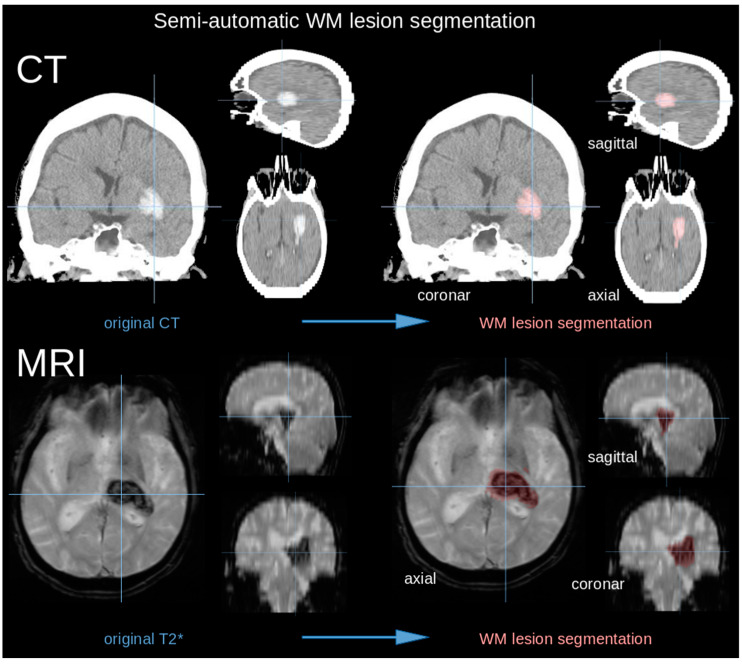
(Upper panel) CT scan in coronar, axial, and sagittal slice representation (see crosshairs) with colored infarct area (right). (Lower panel) MRI scan in axial, sagittal, and coronal slice representation (see crosshairs) with segmented infarct area (right).
